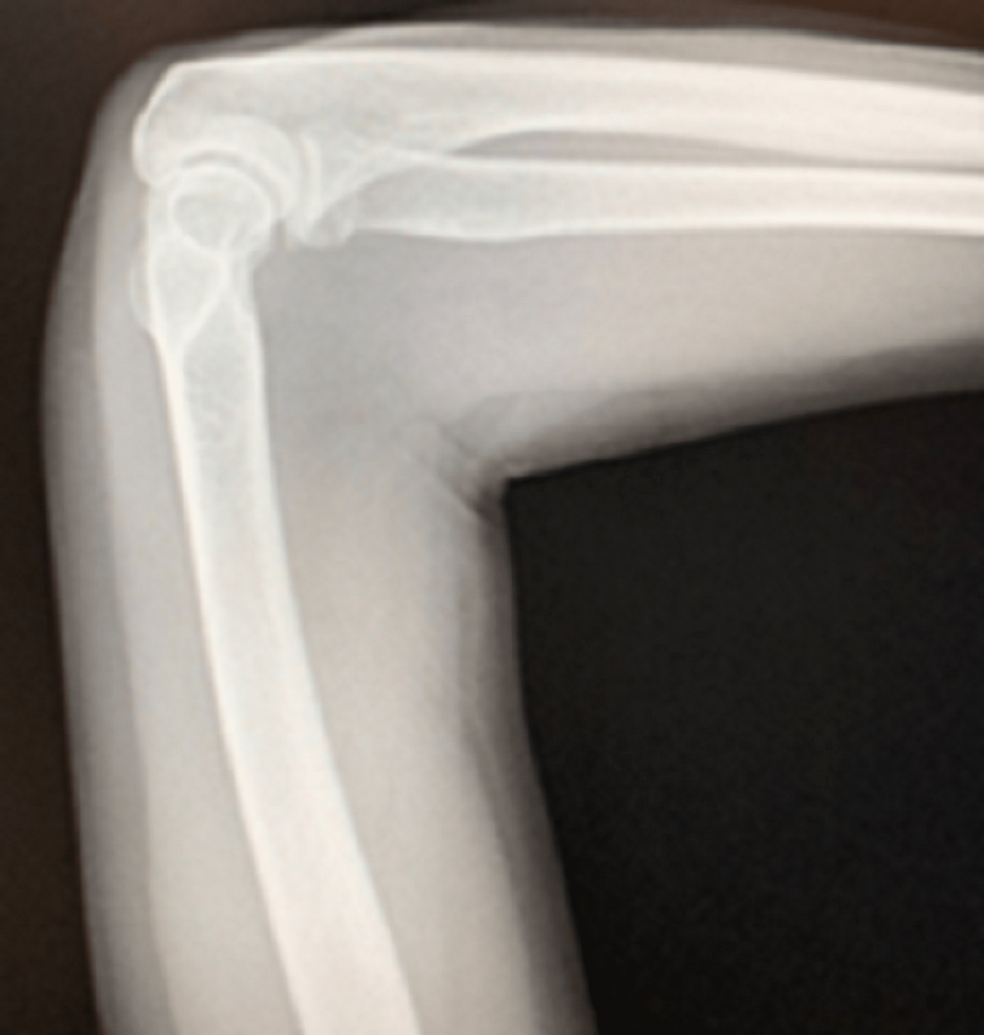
Left elbow radiograph - lateral view Radiograph of the patient’s left elbow in a lateral view. This radiograph does not show any immediate structural defects of the patient’s left radius, ulnar, carpal, and distal humerus bones. Other findings which are present include a possible bone contusion about the left lateral epicondyle.
